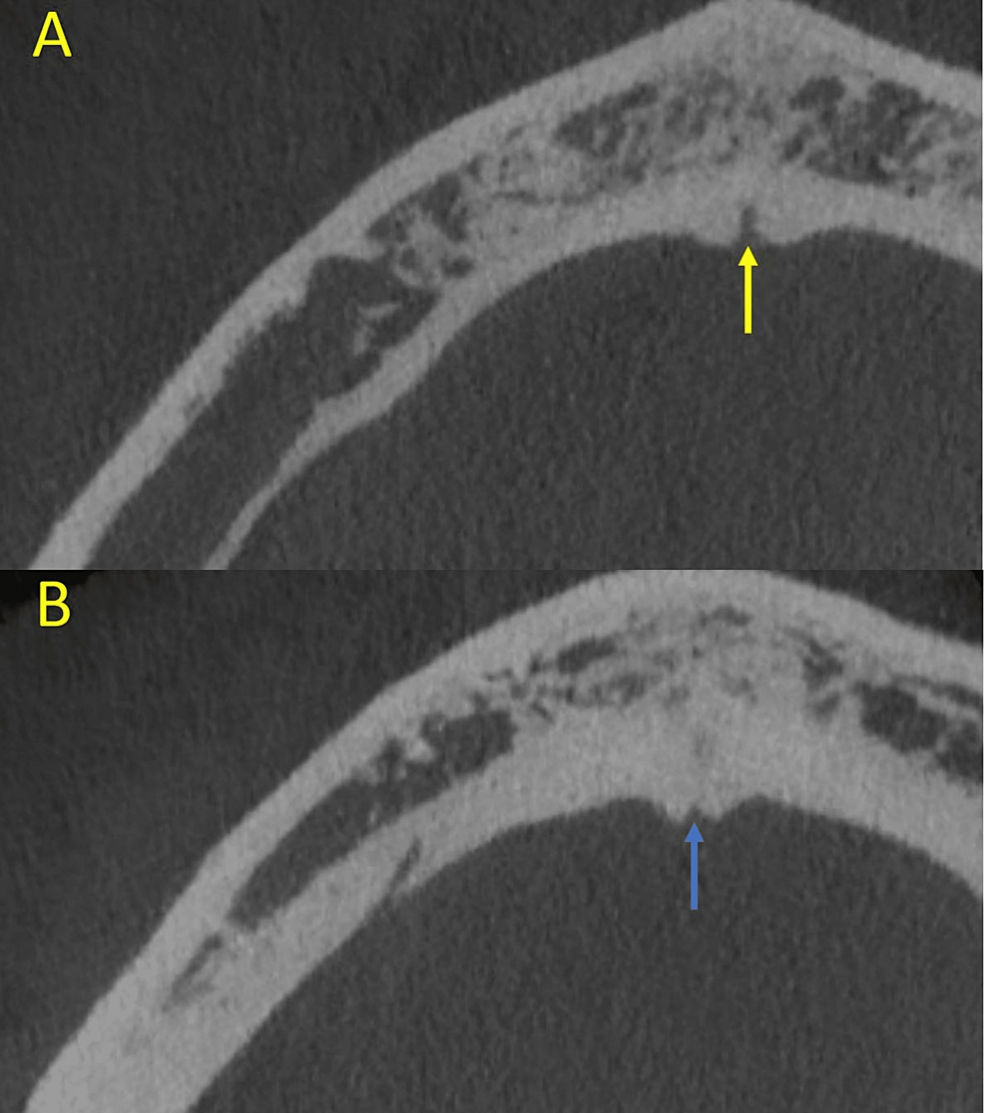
Axial cross-sections of the CBCT scan showing (A) superior level and (B) inferior level of the lingual foramen. CBCT, cone beam computed tomography
